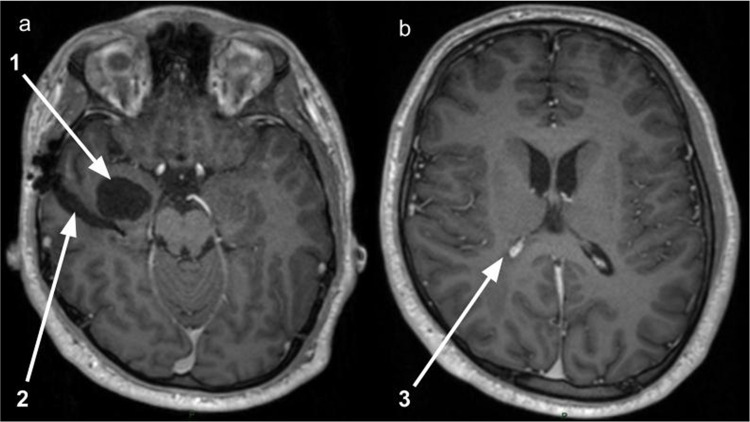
Magnetic resonance imaging (MRI)—post four doses of methotrexate. a Axial T1 + contrast: reduction in size of trapped temporal horn (1), operative tract posterior (2), resolution of leptomeningeal disease. b Axial T1 + contrast: reduction in contrast-enhancing lesion arising from the right choroid plexus (3)
